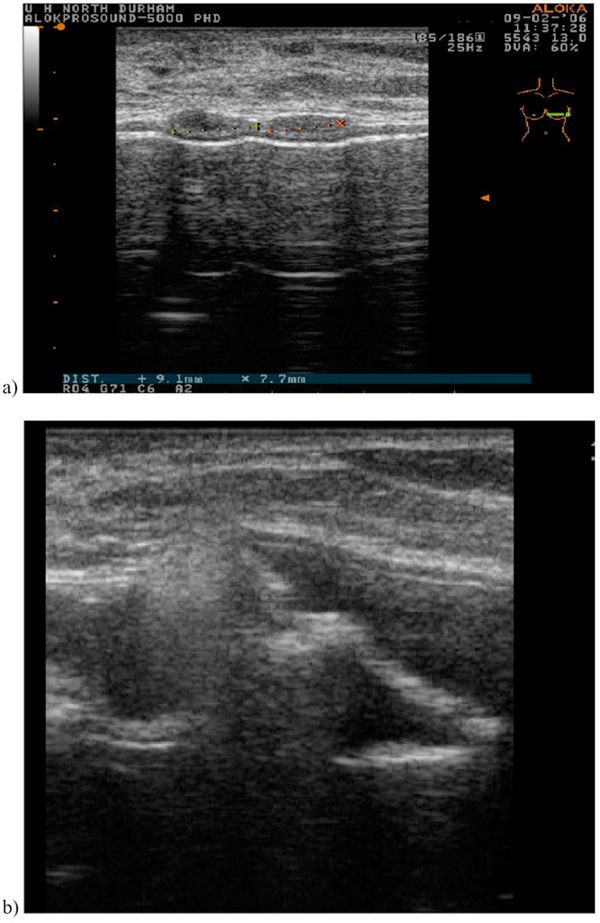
Ultrasound of the left breast implant. Demonstrates several hypoechoic areas measuring 9 and 7mm, respectively, suggestive of extracapsular rupture (a) with gross disorganisation of the internal implant structure in keeping with intracapsular rupture (b).
